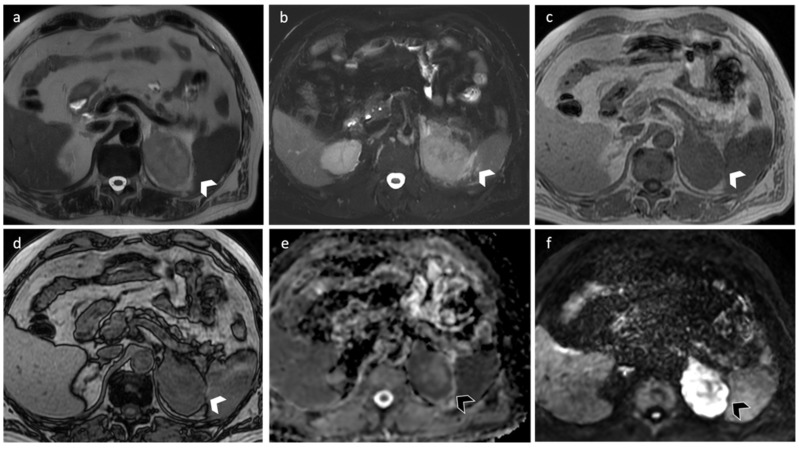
A 67-year-old man with lung cancer. MRI shows a 7 cm nodular lesion on the left adrenal gland characterized by intermediate signal intensity on axial T2-weighted images (a) and perilesional edema (b). The lesion shows intermediate T1-intensity (c) with no signal loss in out-of-phase sequences (d), due to the absence of intralesional fat. DWI (e) and ADC map (f) demonstrated hypercellularity.
